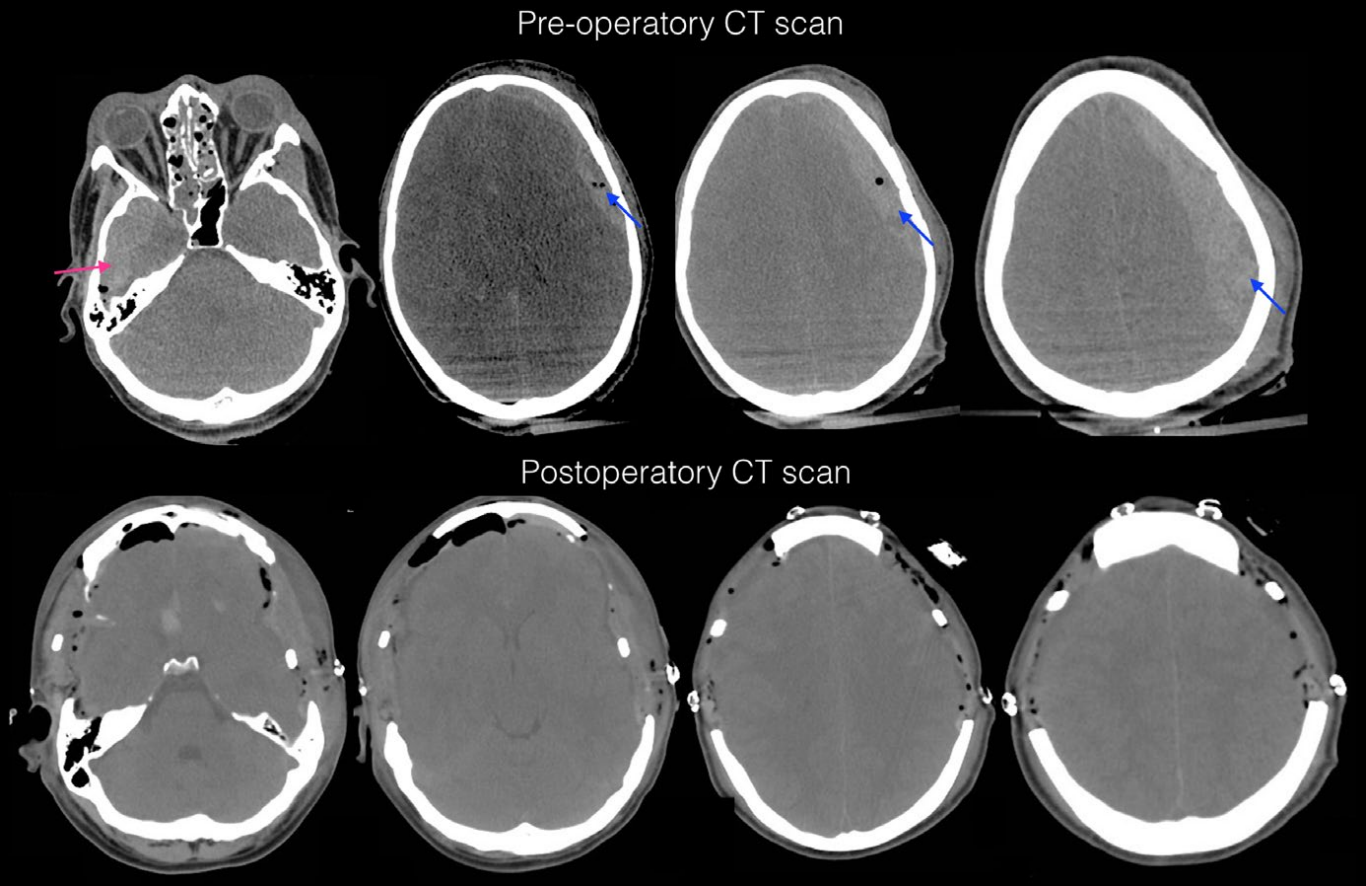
Case 2. Admission CT scan shows a left fronto‐parietotemporal subdural hematoma (blue arrow), a fronto‐basal right contusion, a temporal contusion, a small right temporal epidural hematoma (red arrow), and compressed cisterns. CT scan 24 h after surgery shows a BFTP‐DC. The brain tissue did not herniate through the craniectomies
